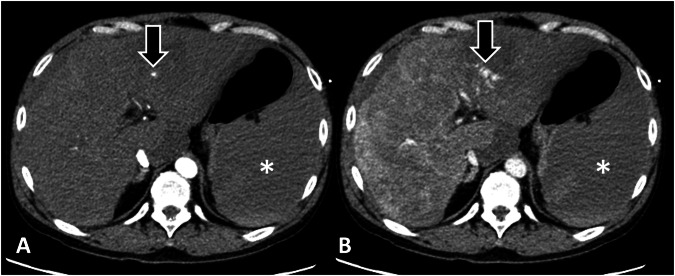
Rapid bleeding and its association with low blood pressures, high packed red cell requirement, and 24-h mortality. Axial CT images in arterial (A) and portovenous phases (B) of a 62-year-old man show a large ill-defined hepatic mass with a focus of active vascular contrast extravasation (AVCE; arrows) and hemoperitoneum (asterisks). The AVCE is very small in the arterial phase but expands quickly in the portovenous phase, having a change in its perimeter between the two phases of 520%, consistent with rapid bleeding. The patient’s initial blood pressure was 92/54 mmHg with a pulse rate of 70 beats/min. He was treated conservatively with medications and 6 units of red blood cell transfusion and passed away within 24 h of admission
